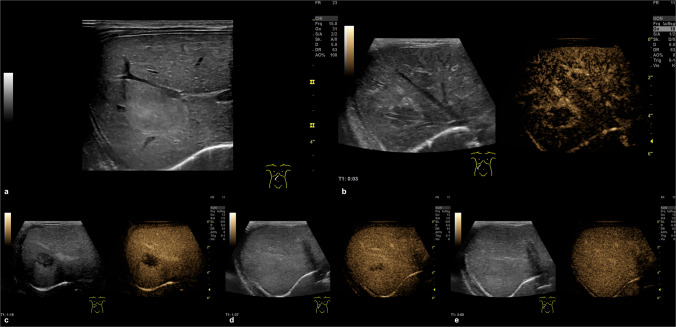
Infantile single low-flow hepatic hemangioma in a neonate born at 40 ^1^/_7_ weeks’ gestation, identified incidentally on the 11th day of life (weight 2.7 kg, length 50 cm), who was transferred to our clinic. a Subcostal view.B-Mode image of an echogenic, roundish, and sharply delineated lesion in the right liver lobe of 2.4 × 1.7 × 1.9 cm. Even with high sensitivity Doppler or microvascular imaging no vessels could be detected in the lesion (not shown). b Early arterial phase, 3 s post-injection: nodular rim enhancement in the periphery of the lesion, early non-enhancement of the center. c Portal venous phase, 1 min and 19 s post-injection: nodular rim enhancement in the lesion, slow centripetal fill-in. d Portal venous phase, 1 min and 37 s post-injection: slow further centripetal fill-in within the lesion. e Late phase, 3 min after post-injection. Complete fill-in in the whole lesion, isoenhancement of the lesion compared with the liver parenchyma, no wash-out. Typical finding for an infantile low-flow hemangioma, which is far less common in infants than infantile high-flow hemangiomas (“iris diaphragm phenomenon”). Because of the classical finding no further diagnostic imaging or biopsy was needed. Spontaneous regression in follow-up scans
